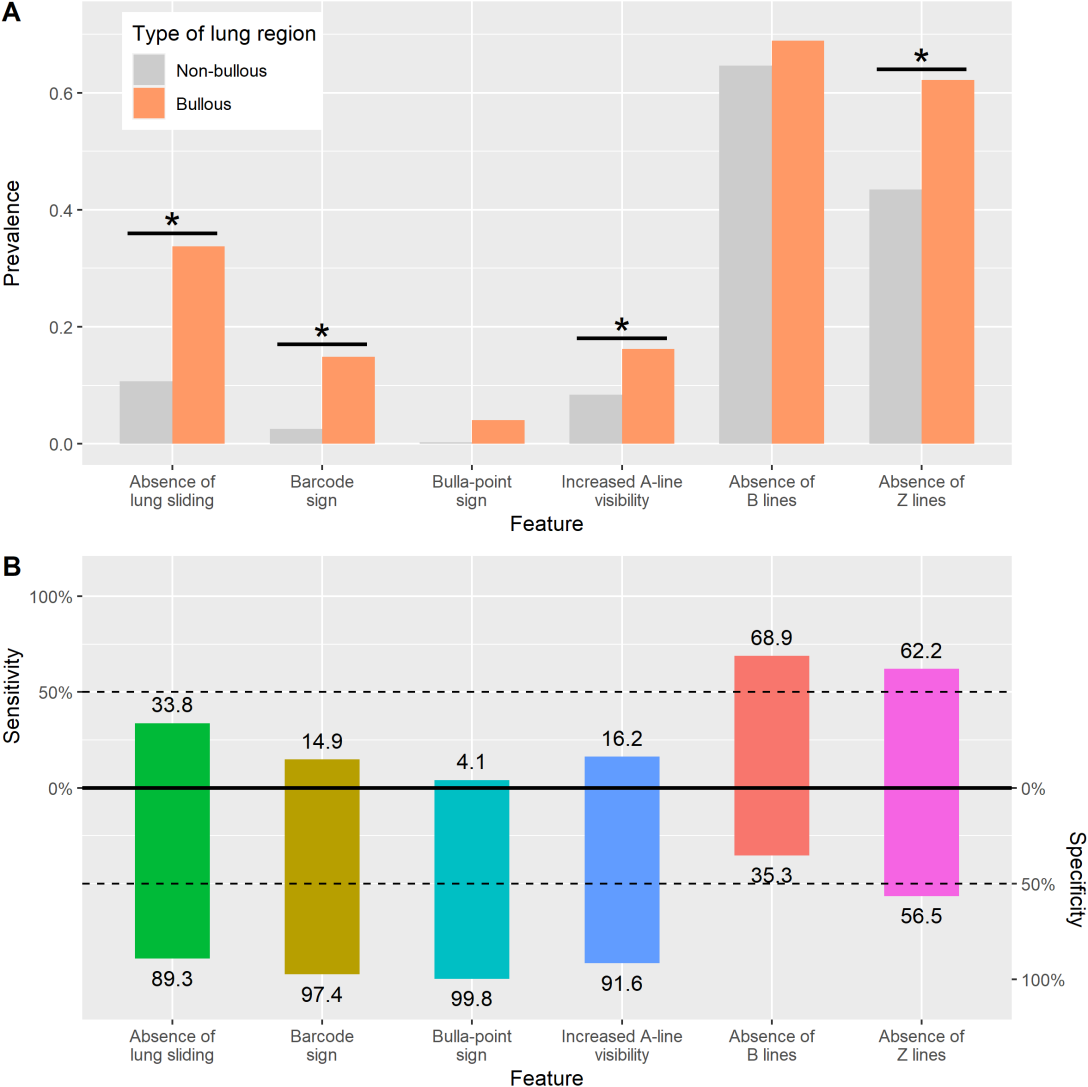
Comparison of LUS features between bullous and non‐bullous regions. (A) Bar plot of the prevalence of six sonographic features in regions with bullous emphysema with at least two intercostal spaces of pleural contact (bullous regions) and without (non‐bullous regions). Asterisks indicate statistical significance (*p* < 0.05). (B) Representation of the overall diagnostic performances of each sonographic feature for the positive diagnosis of the bullous region. Sensitivity is displayed on the upper part of the vertical axis, while specificity is shown on the lower part.
